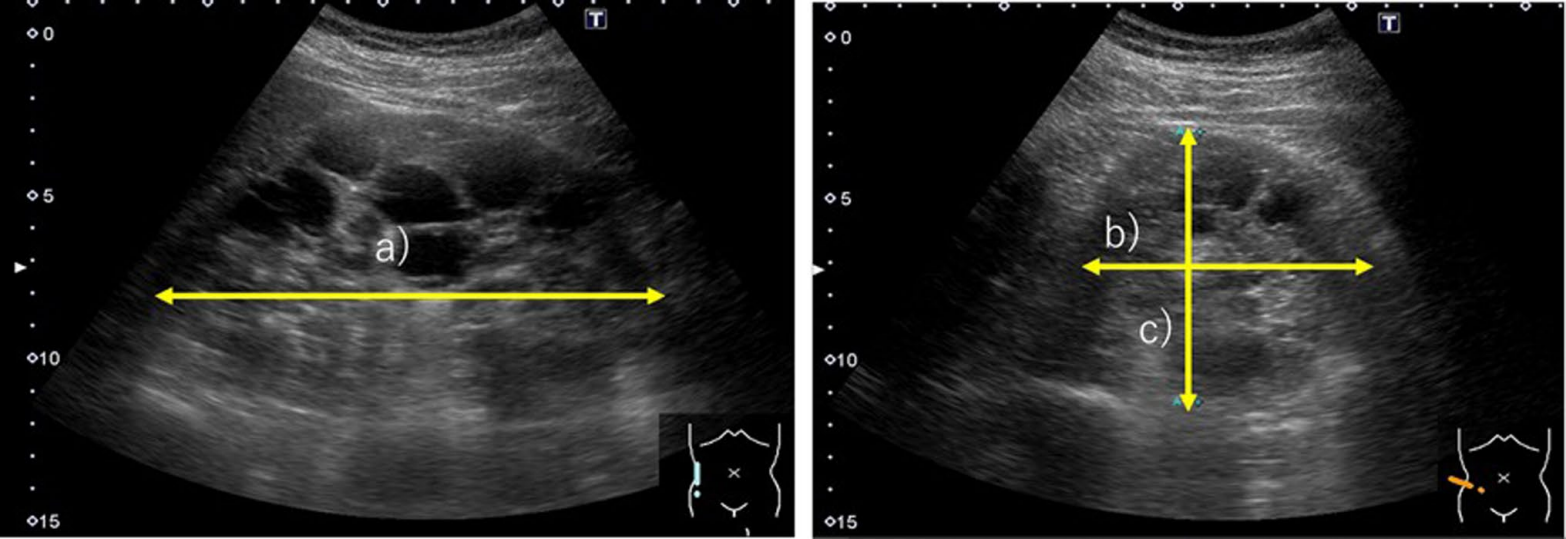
Measurement of kidney size using ultrasonography in a male patient in his 20 s. a. Maximum renal height (length): 151 mm. b. Maximum anteroposterior distance: 82 mm. c. Maximum mediolateral distance: 64 mm. TKV was 415 mL
